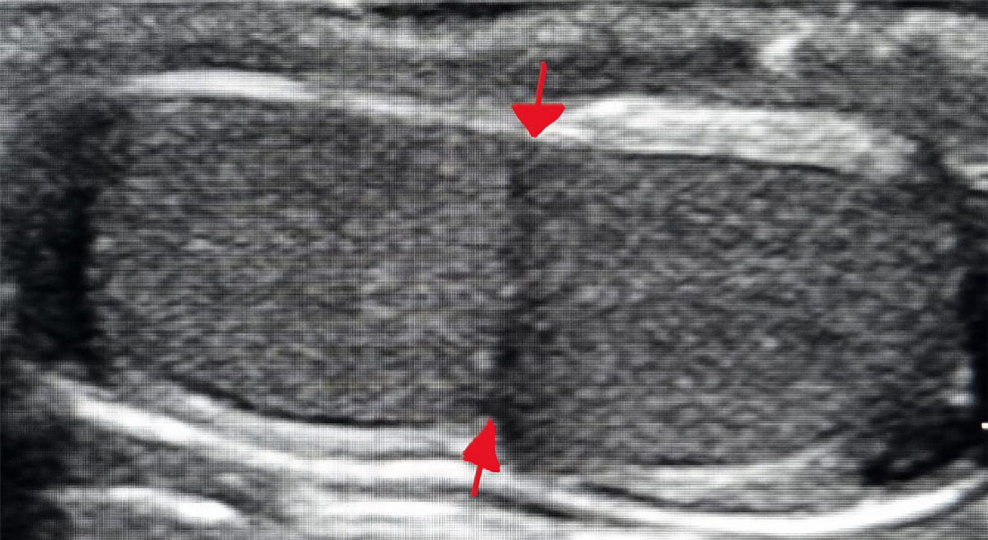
Transverse ultrasound showing a right bilobed testicle (red arrow shows the separation of the 2 lobes).
